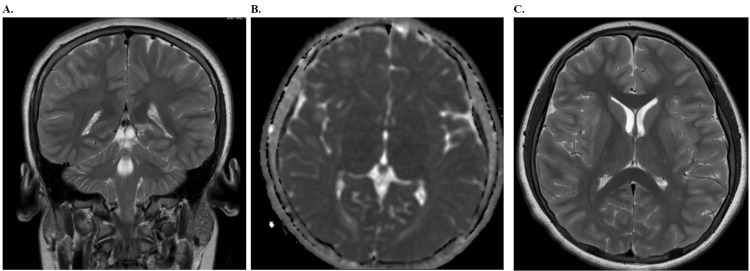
MRI characterization of white matter involvement in myelin oligodendrocyte glycoprotein antibody disease (MOGAD) in a pediatric patient A. Coronal T2-weighted image demonstrates extensive hyperintensity in the deep white matter, indicative of demyelination B. Axial diffusion-weighted imaging (DWI) shows areas of restricted diffusion, suggestive of acute inflammation C. Axial T2-weighted image reveals symmetric hyperintense lesions in the periventricular white matter, consistent with active demyelination
